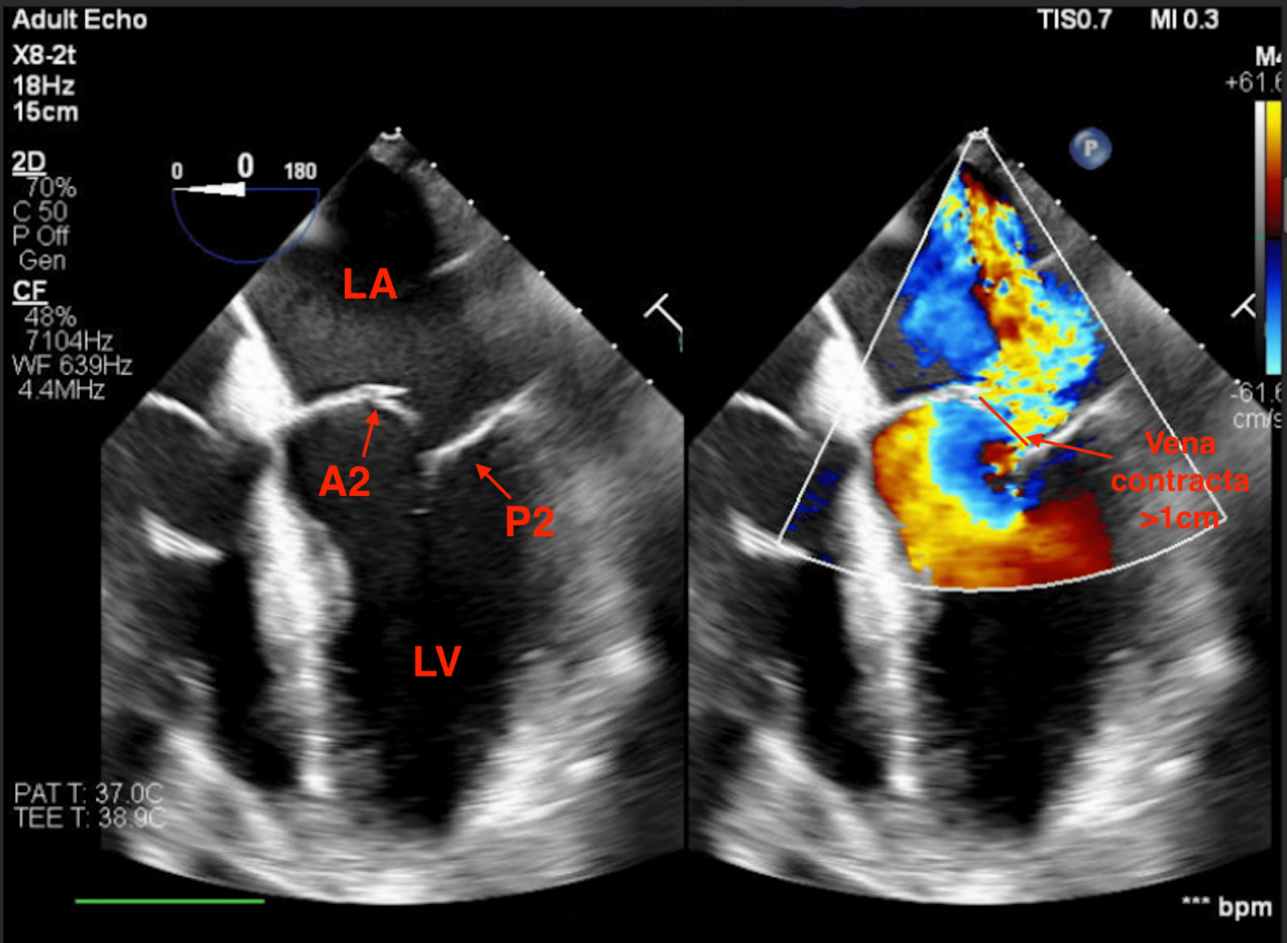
TEE, four-chamber view, showing severe MR A2, anterior mitral leaflet 2 segment; LA, left atrium; LV, left ventricle; MR, mitral regurgitation; P2, posterior mitral leaflet 2 segment; TEE, transesophageal echocardiogram
